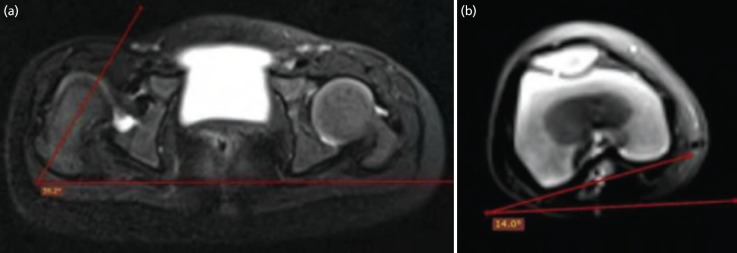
Femoral anteversion (FA) angle: Axial T2-weighted fat-suppressed image of the hip with right-sided DDH in a 1-year-old female child. (a) The α angle (59.2°) is the angle between the line parallel to femoral neck and the horizontal reference line. (b) The ß angle (14°) is between the line through the posterior ipsilateral femoral condyles and the horizontal reference line. The FA angle is corrected (α -ß) and it is calculated as 45.2°.
